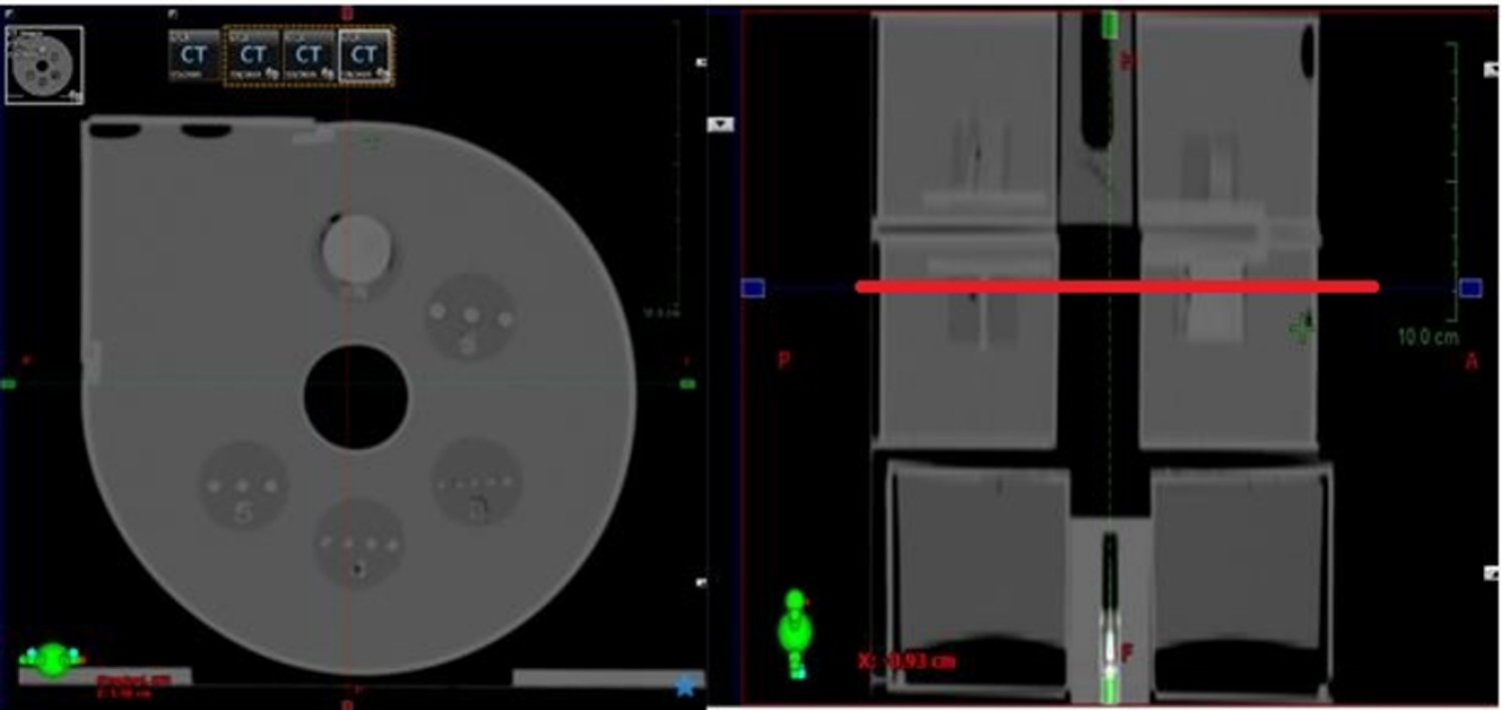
CT image of the low-contrast detectability slab.
